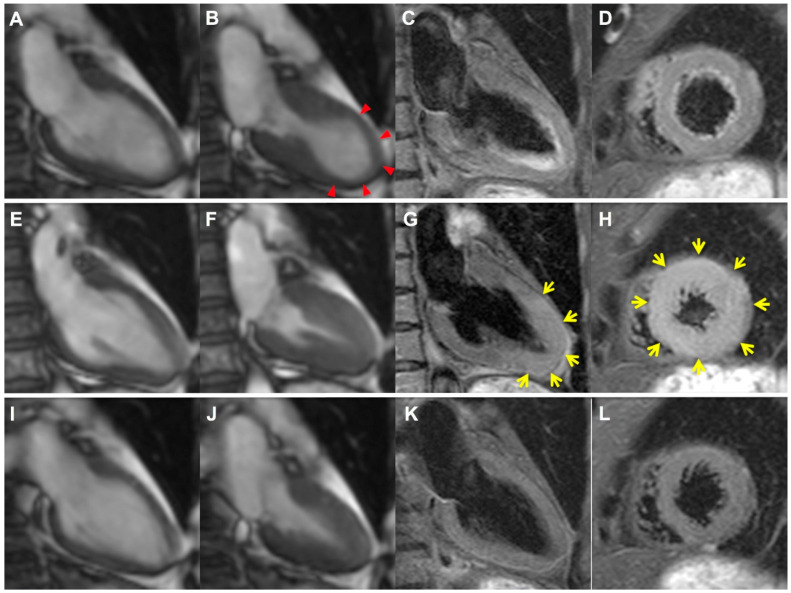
Serial change of myocardial edema in a representative patient with TTS. In the acute phase, apical ballooning was observed ((A,B), red arrows). Myocardial edema on T2-weighted images was slight (C,D). In the subacute phase, wall motion was completely recovered (E,F), whereas more thickened apical wall with more severe myocardial edema was observed compared with the acute phase ((G,H), yellow arrows). In the chronic phase, the final CMR imaging demonstrated normal systolic function (I,J) without apical wall thickening or myocardial edema (K,L). CMR, cardiovascular magnetic resonance; TTS, takotsubo syndrome. Adapted with permission from ref. [13]. Copyright 2017 the Japanese Circulation Society.
